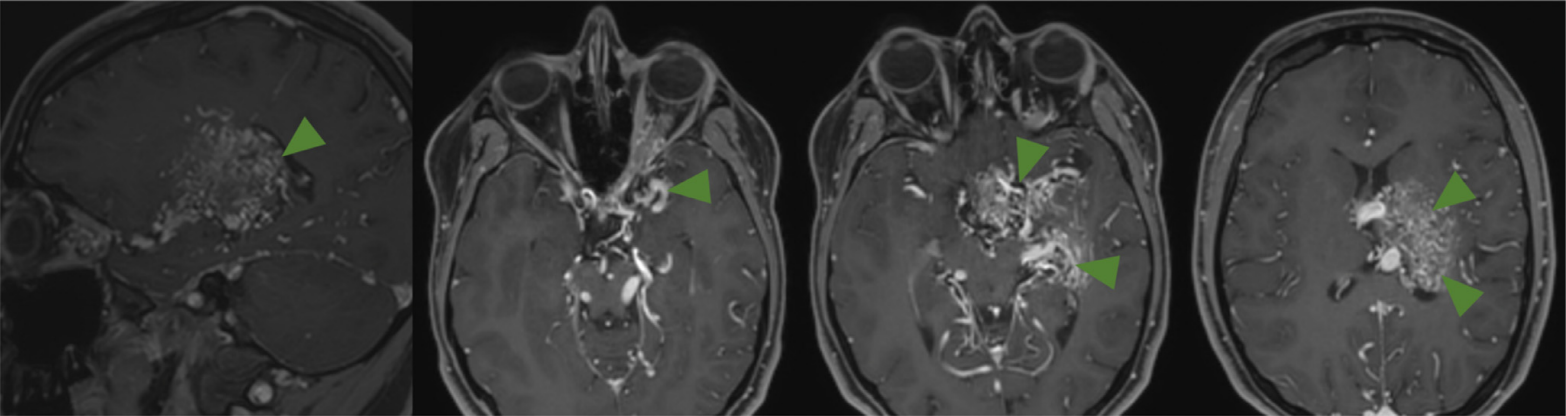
Postcontrast MRI brain at (A) sagittal and (B-D) axial cuts demonstrating the extent of the AVM (green arrows).
